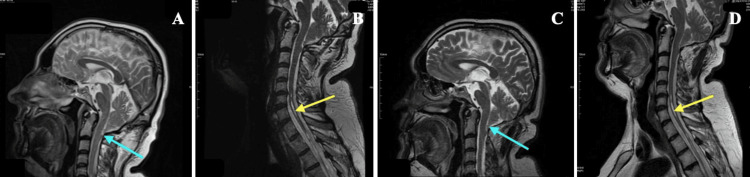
The MRIs of the patient Preoperative sagittal T2-weighted MRI of the brain shows 9-mm tonsillar descending below the foramen magnum (cyan arrow) (A); Preoperative sagittal T2-weighted  MRI of the cervical spine shows cervical syringomyelia (yellow arrow) (B); Sagittal T2-weighted MRI of the brain at second postoperative year (cyan arrow: showing tonsillar descending below the foramen magnum) (C);  Sagittal T2-weighted  MRI of the cervical spine at second postoperative year shows no changes in cervical syringomyelia (yellow arrow) (D).
